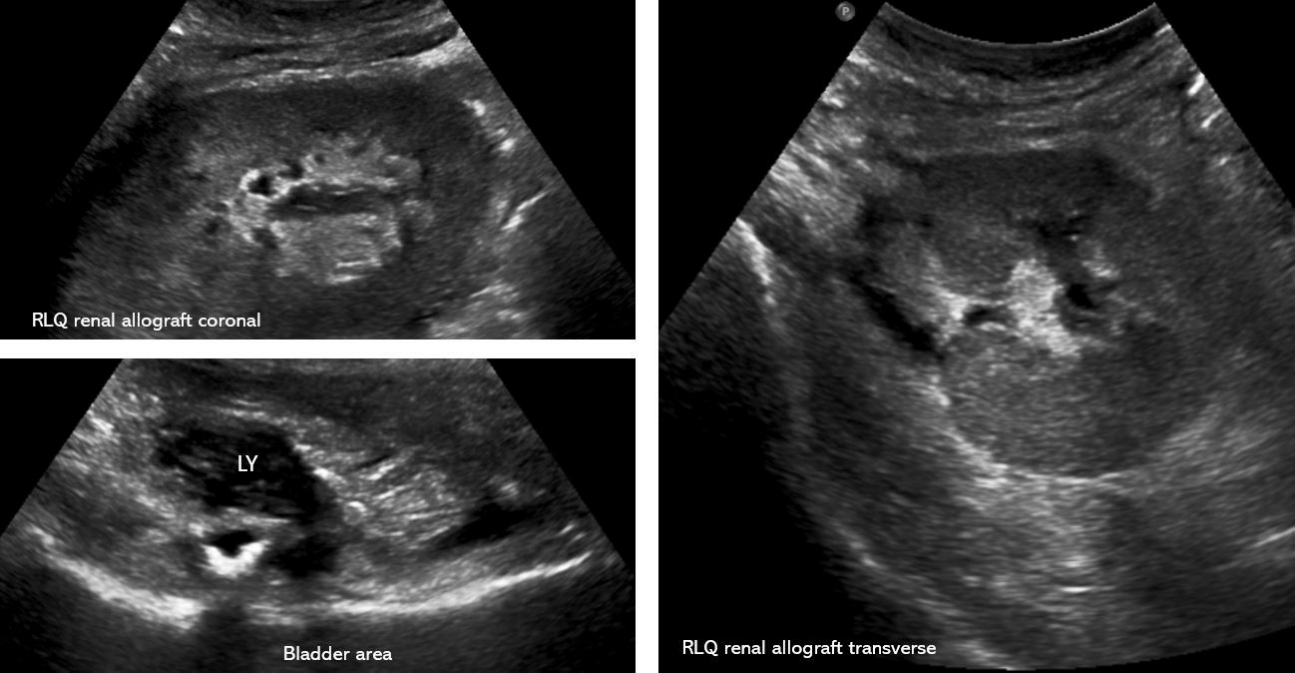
Repeat sonogram after the intervention demonstrating significant improvement in hydronephrosis and lymphocele.
